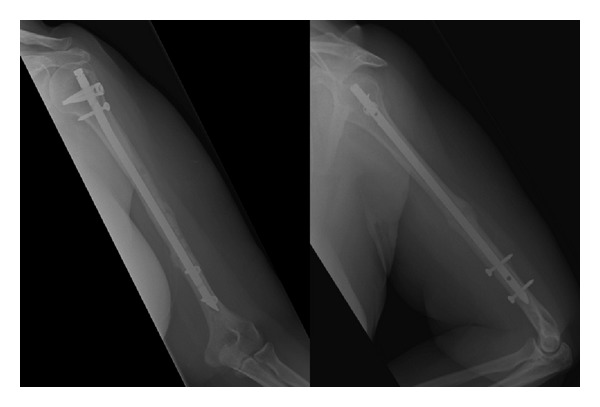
AP and lateral radiographs showing fracture union following revision to intramedullary nail.
